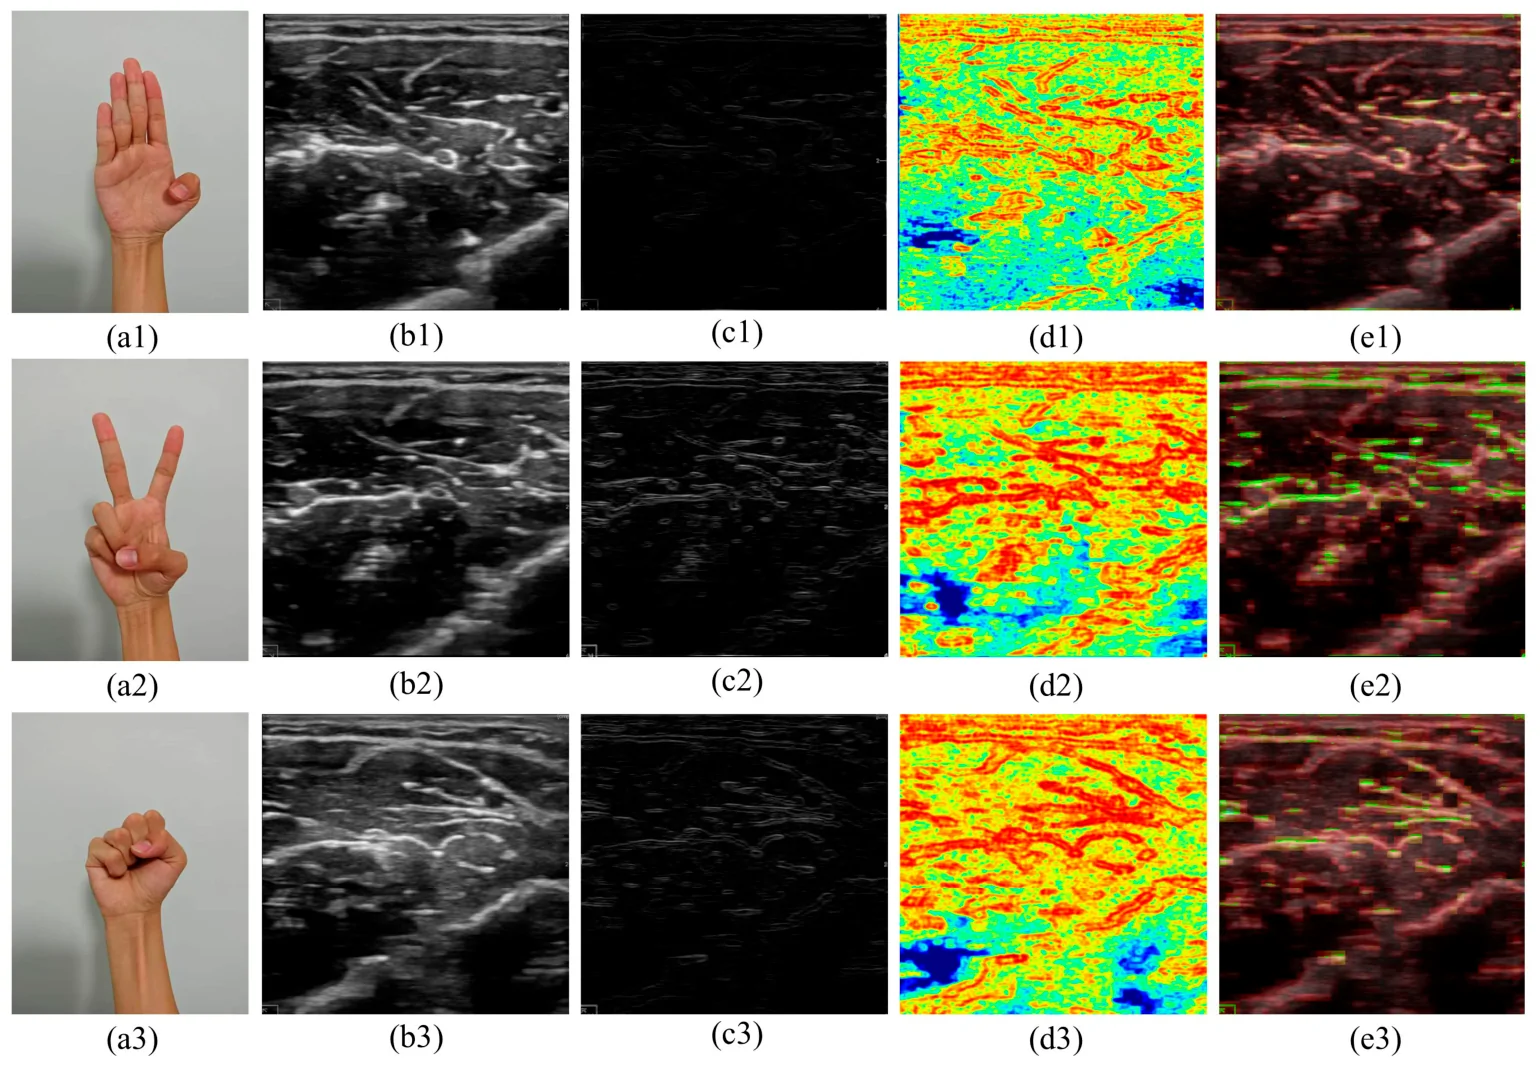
Visualization results of feature extraction for Gesture 01, 09, and 13 from Subject 2. (a) Gesture images; (b) Raw ultrasound frames of the gesture; (c) Gradient maps of the gesture; (d) Entropy heatmaps of gesture; (e) Feature fusion maps of the gesture.
